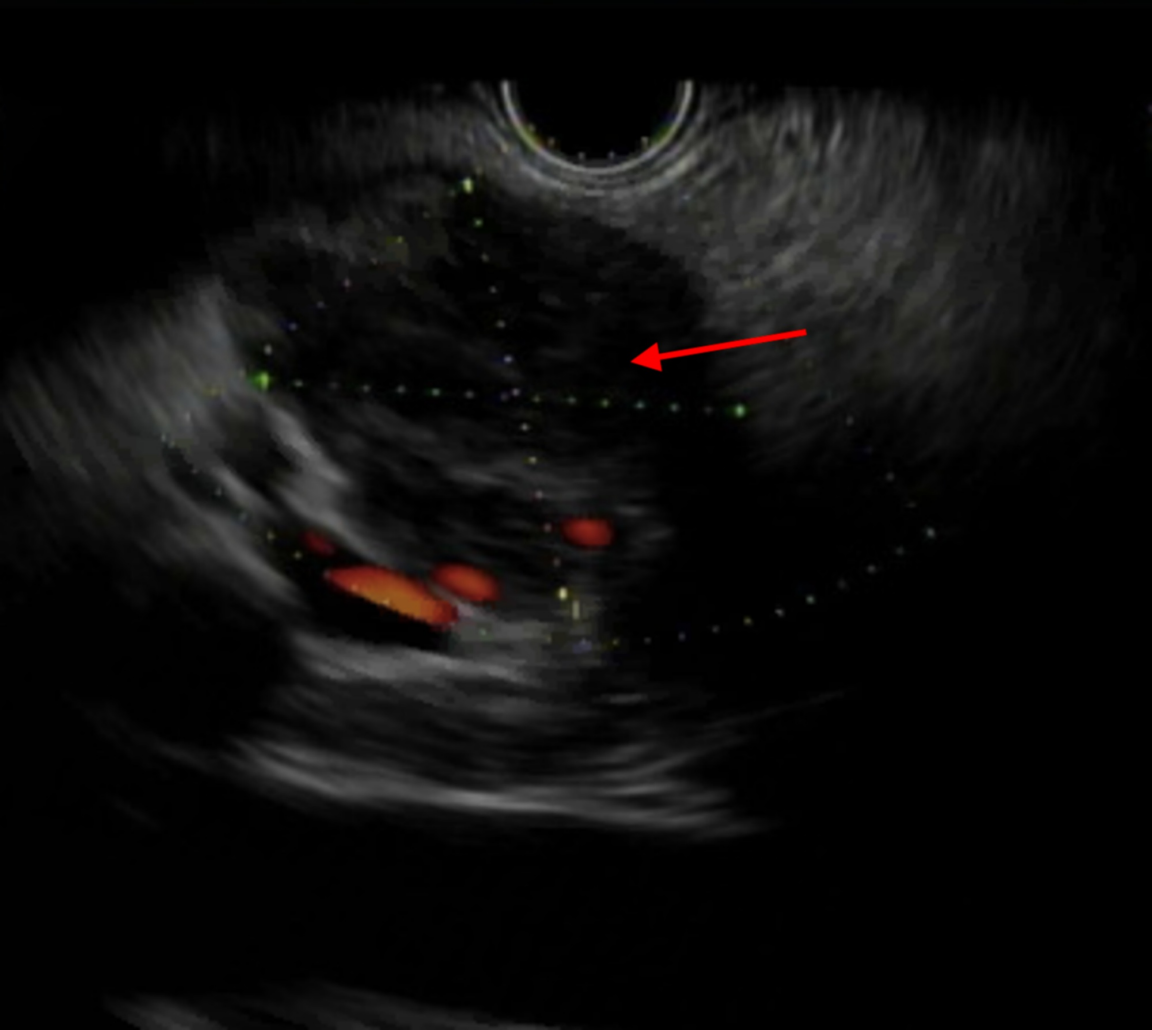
EUS demonstrating 30 mm x 26 mm hypoechoic lesion. EUS, endoscopic ultrasound
